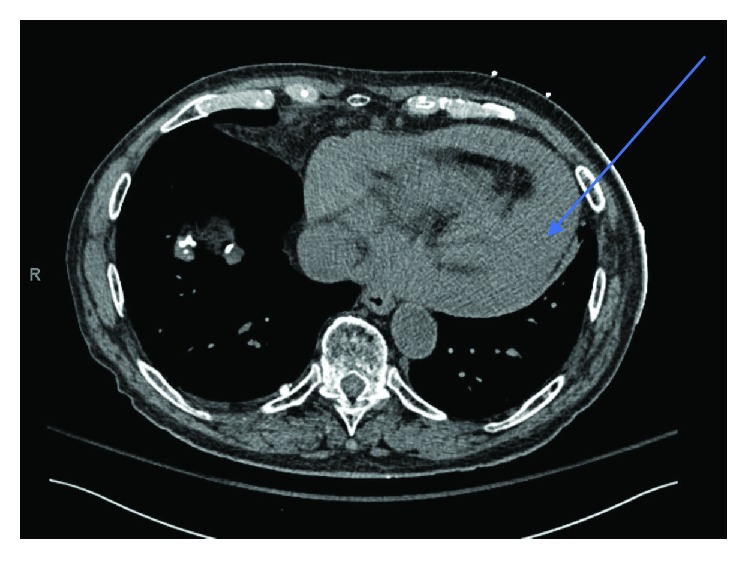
Chest CT scan (transverse view) showing fluid in the pericardial space.
